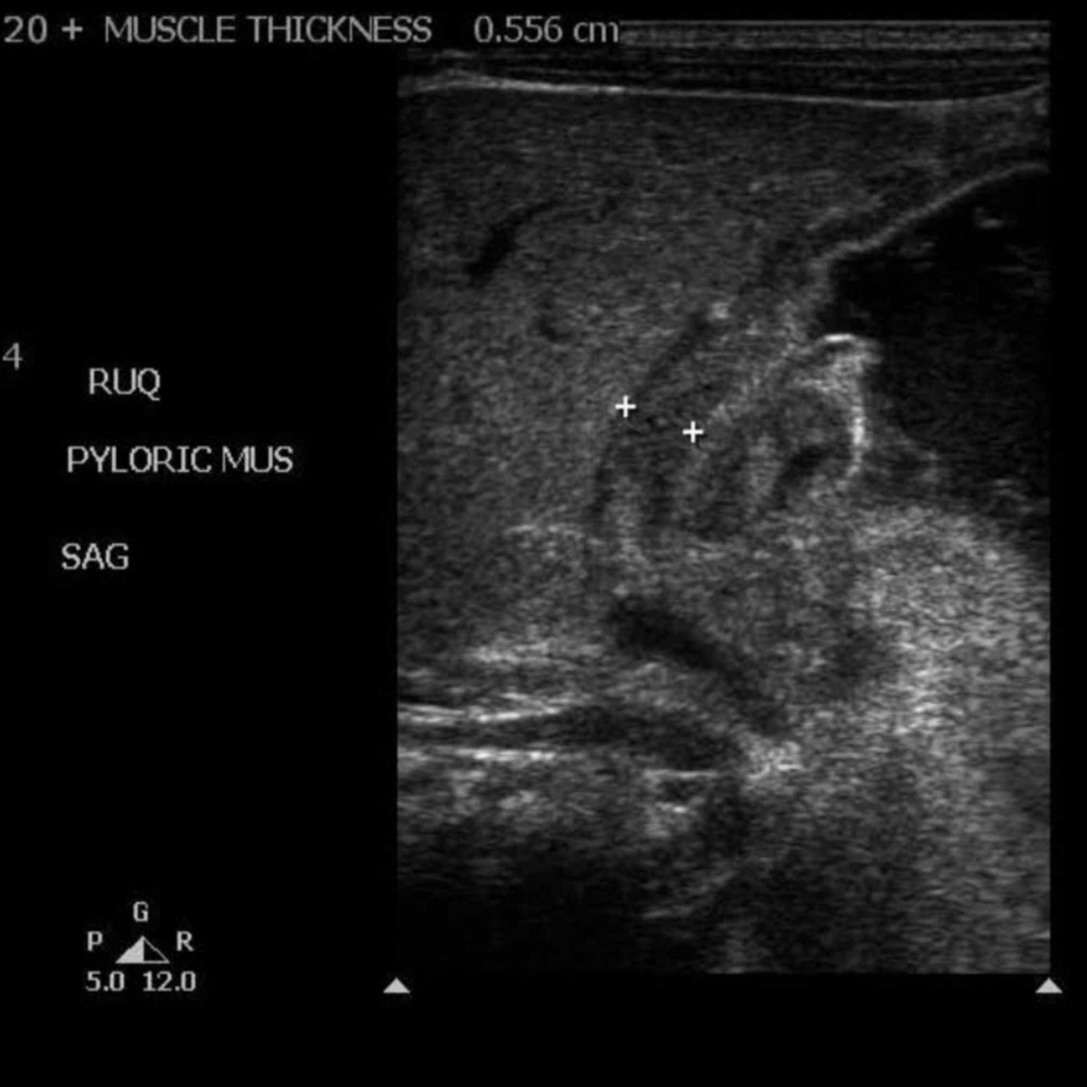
Ultrasound image showing the ingested material in the gastric antrum and pyloric stenosis US: ultrasound; SAG: sagittal plane; pyloric mus: pyloric muscle; RUQ: right upper quadrant
